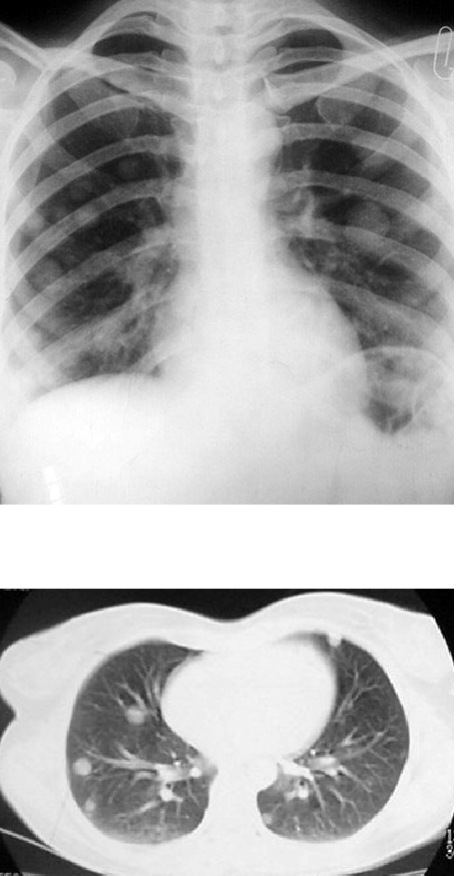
Chest X-ray and Computerized tomographic scan showing multiple metastatic nodules in the lung
